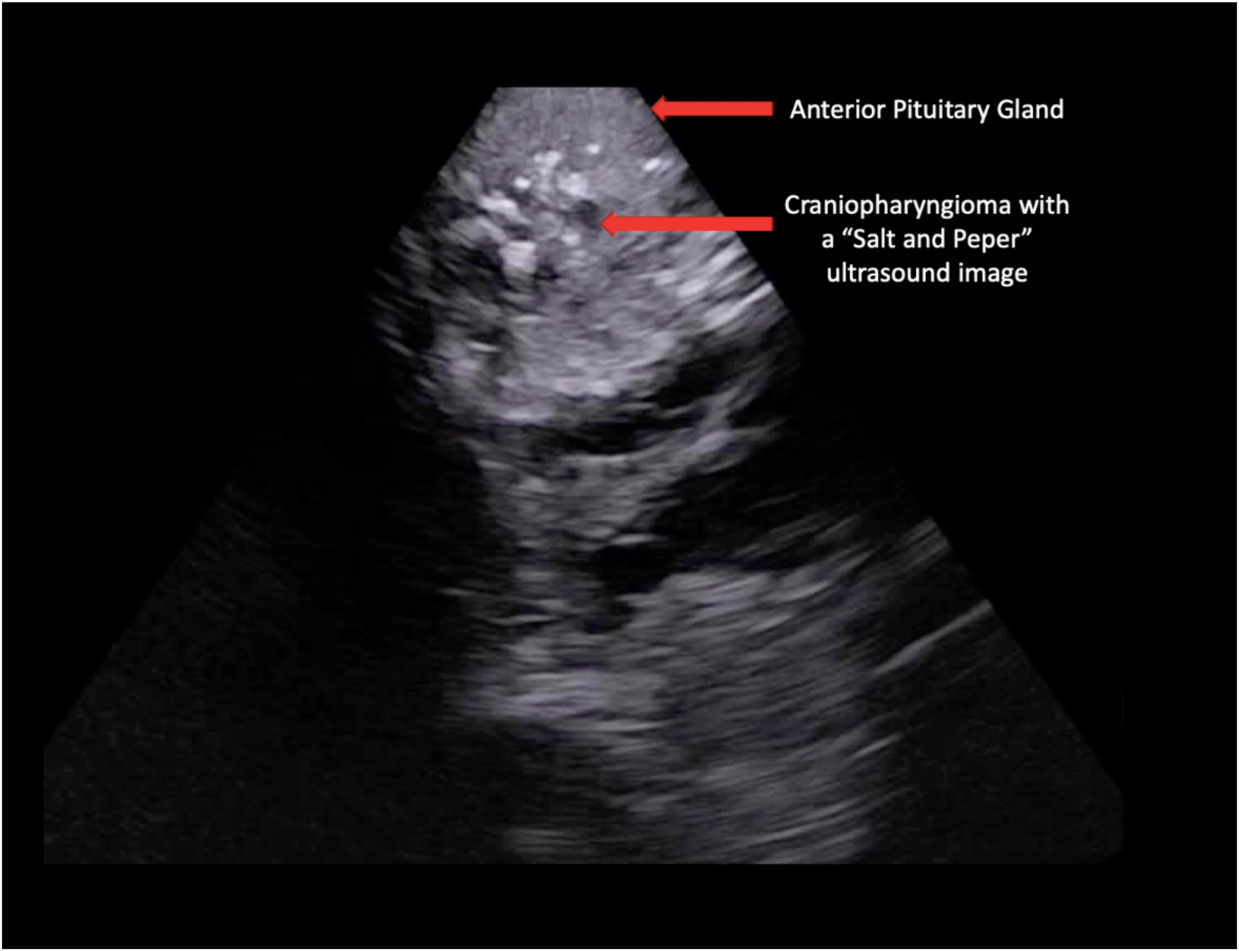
Endonasal sonographic pattern of a craniopharyngioma; intraoperative US picture demonstrating the sonographic characteristics of the craniopharyngioma and its relationship to the anterior pituitary gland.
